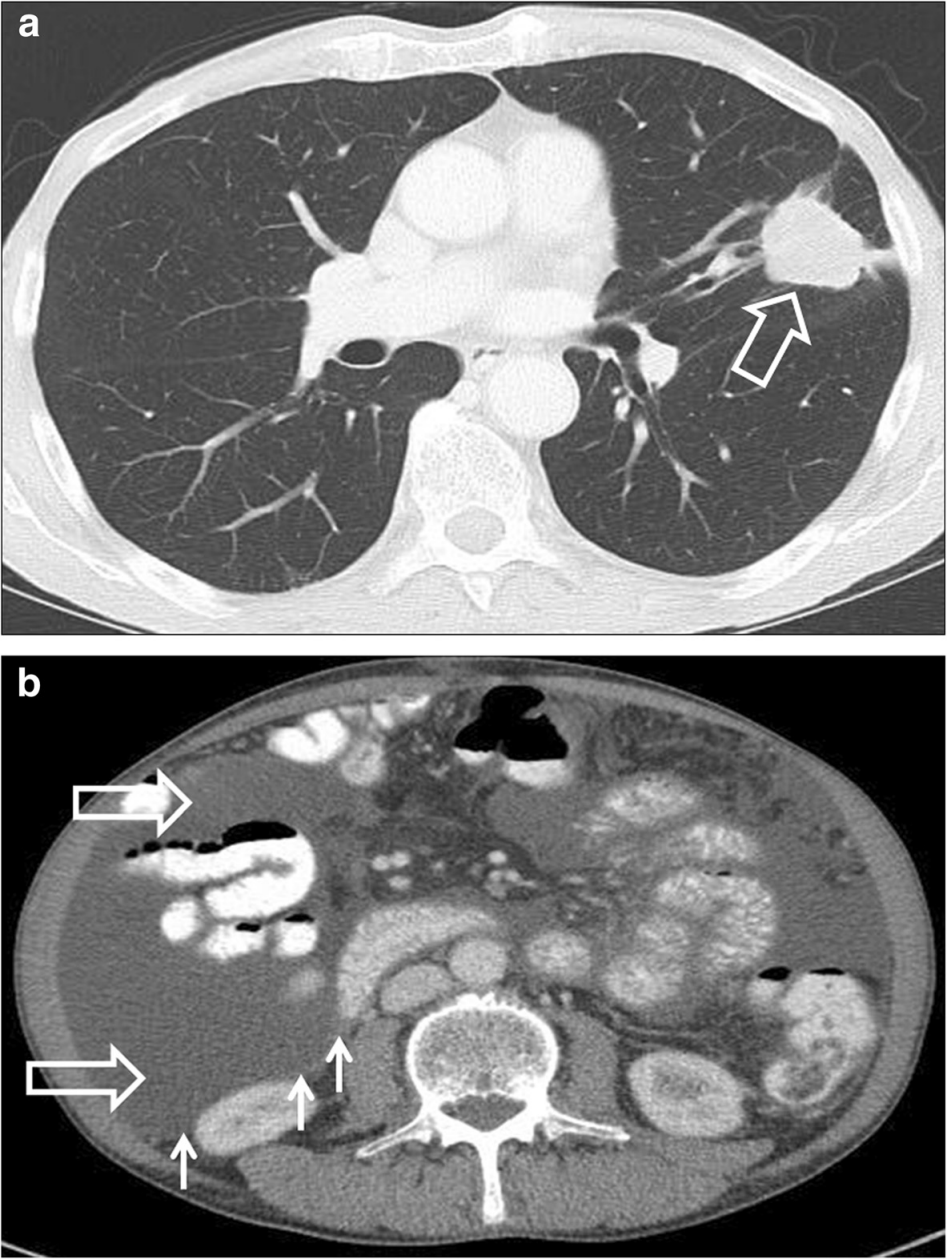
Contrast-enhanced computed tomography scan of the chest and abdomen showing disease progression in June 2011. (a) A 3.3 × 2.8 cm mass (big arrow) in the left lung and (b) ascites in all 4 quadrants of the abdomen (big arrow) and peritoneal nodules (small arrows).
